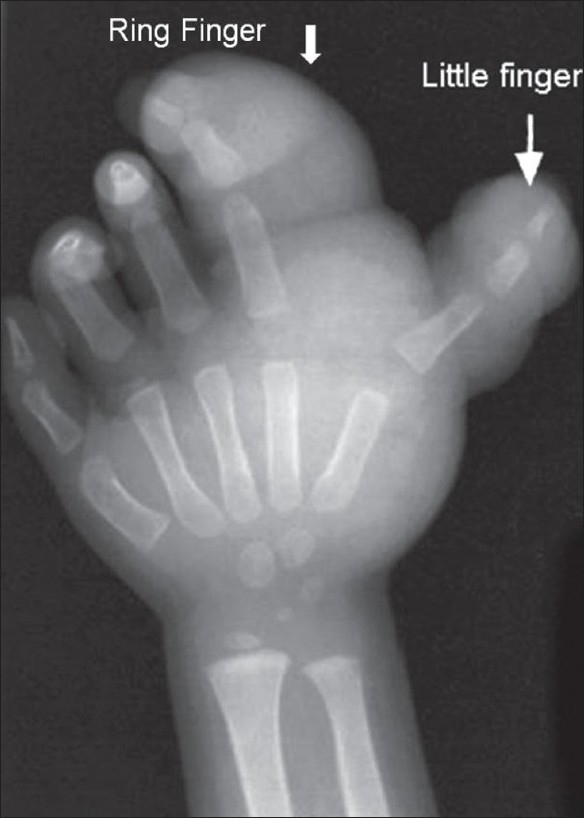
Case 2: Plain radiograph of the left hand shows soft tissue swelling (arrows) causing enlargement of the ring and little fingers, along with dorsal angulation of the former and lateral angulation of the latter
